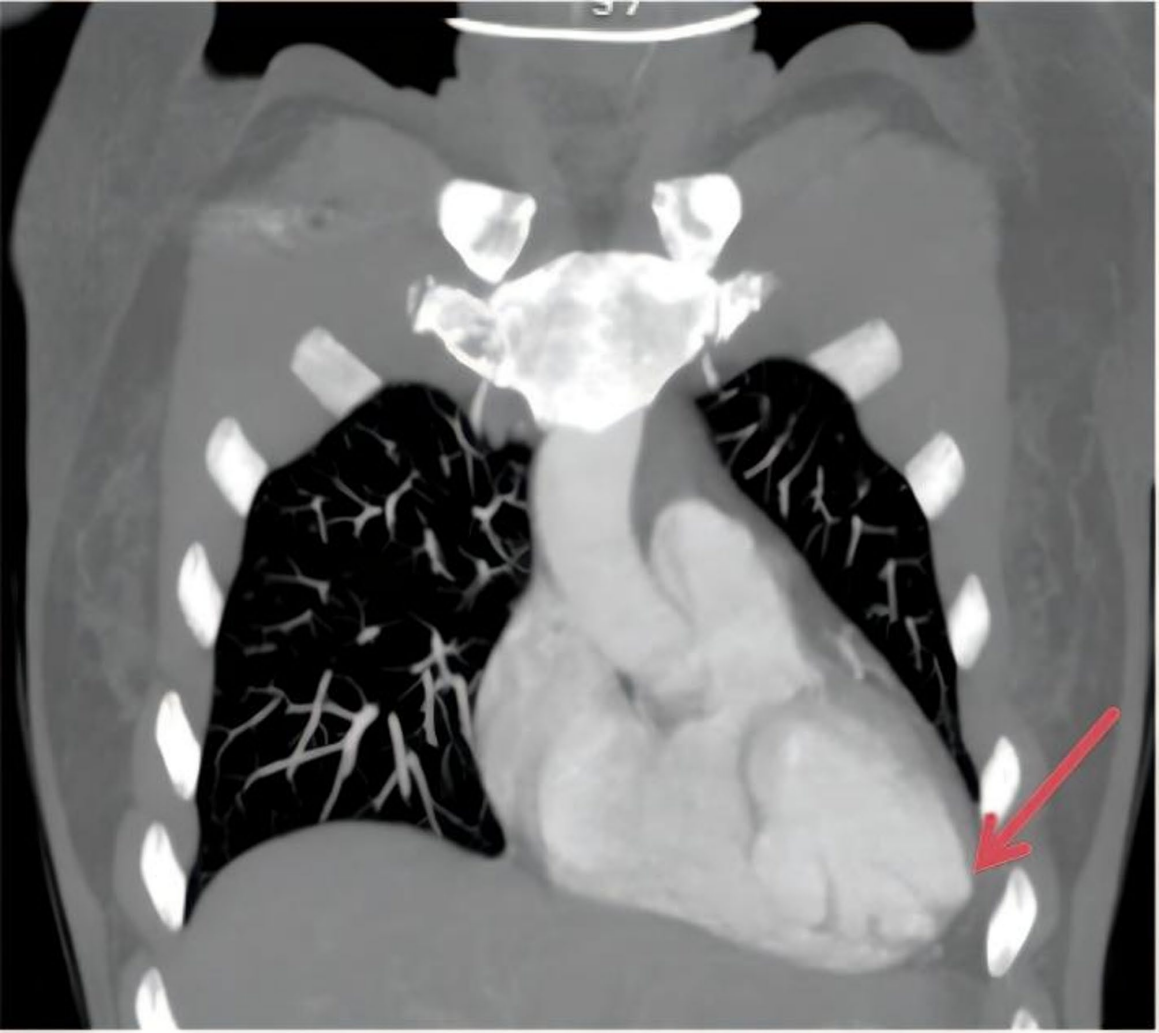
CTA of the thoracic aorta revealed ventricular septum-inferior wall aortic dissecting aneurysm formation.Multiple cystic protrusions were present in the interventricular septum and inferior wall, and no contrast agent overflow was observed in the pericardial cavity
